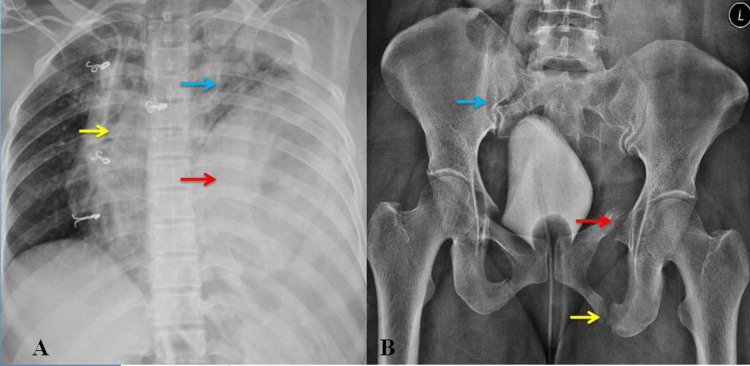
(A) Chest x-ray showing a shift of the mediastinum towards the right (yellow arrow), the collapse of the left lung (blue arrow), and herniated stomach in the thorax (red arrow); (B) Pelvis x-ray showing fractures of the right sacroiliac joint (blue arrow), left superior pubic ramus (red arrow), and inferior pubic ramus (yellow arrow).
